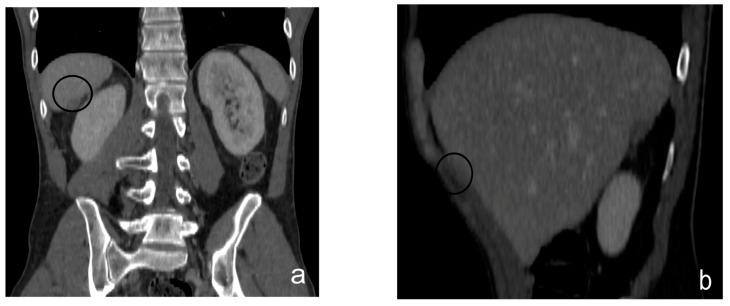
Contrast-enhanced CT scan, coronal view (a) and sagittal view (b). Nodule of peritoneal carcinosis (black circle), localized on the Glissonian surface.
